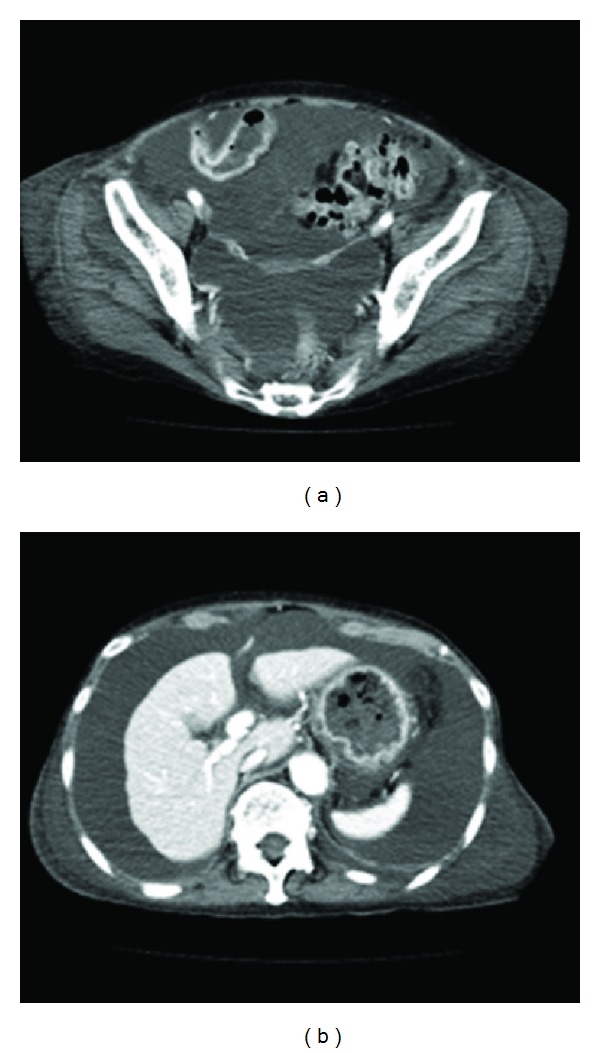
Plain abdominal CT. (a) Multiple sigmoid diverticula. (b) A large amount of ascitic fluid. No evidence of perforation was found.
